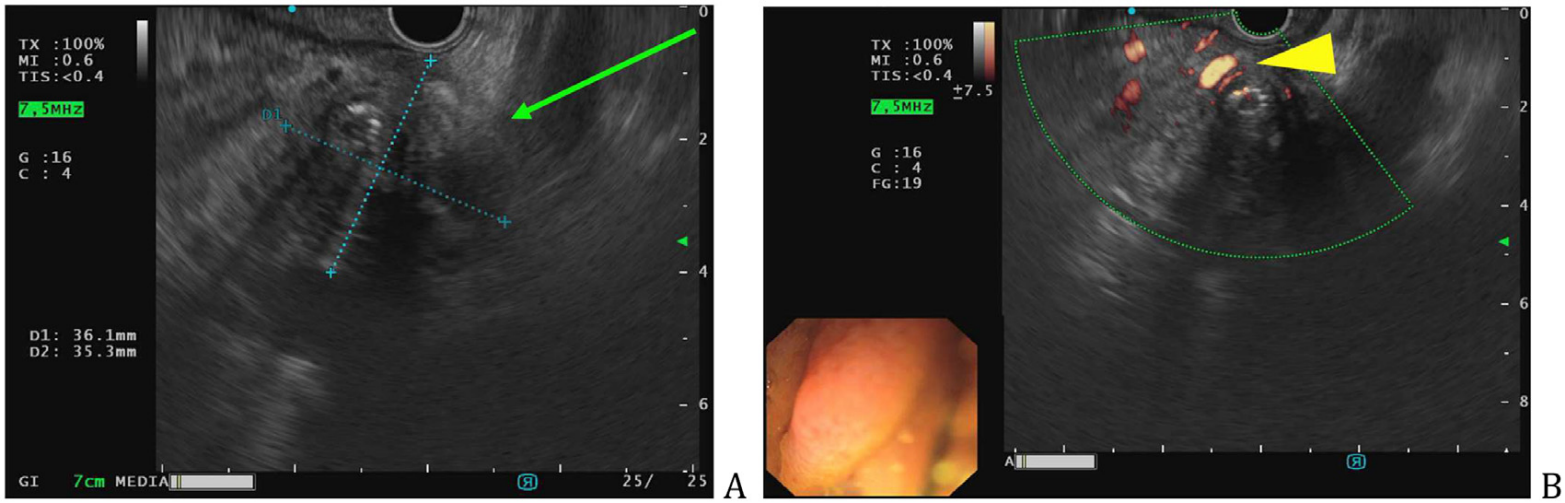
Endoscopic ultrasound showing pathological mass around the pancreatic head (green arrow) (A), with signs of peripheral blood flow (yellow arrowhead) (B).
